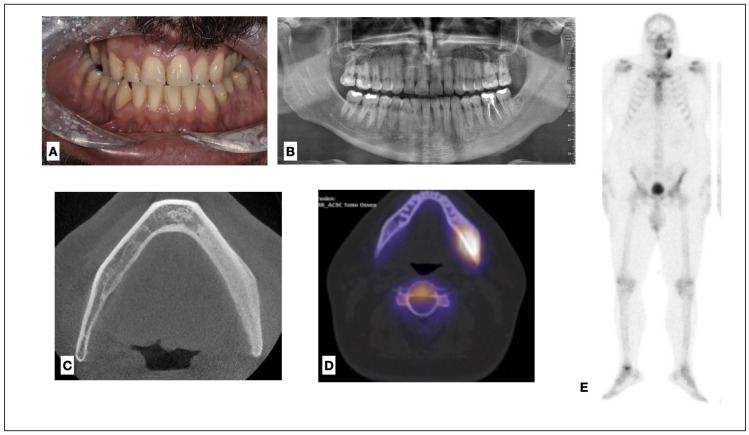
Intraoral view (A). Dental panoramic radiograph: a slight increase in the bone density of the left hemimandible can be noted (B). CT scan in axial view of the mandible, with marked sclerosis of the left hemimandible (C). Axial view of the scintigraphy, with a marked hyperuptake of the left mandible (D). The total body scintigraphy does not show any other areas of involvement (E).
